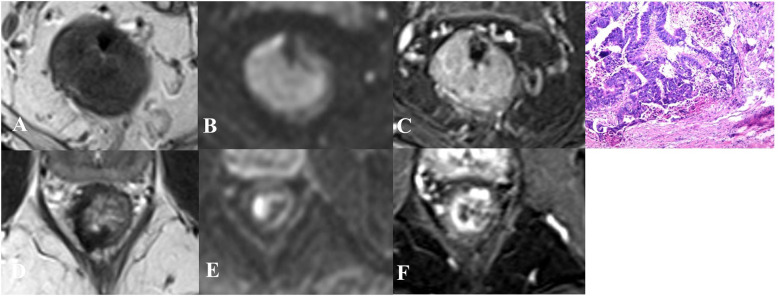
Imaging and pathological findings in a 71-year-old male with low rectal adenocarcinoma. (A–C) Baseline axial T2-weighted imaging, diffusion-weighted imaging (DWI), and contrast-enhanced MRI show a tumor occupying &gt;3/4 of the bowel circumference, characterized by intermediate T2 signal, restricted diffusion, and contrast enhancement. (D–F) After neoadjuvant chemoradiotherapy, the lesion area exhibits thickened, hypointense changes on T2-weighted imaging, persistent restricted diffusion on DWI, and rigid, thickened enhancement on contrast- enhanced imaging. (G) Postoperative pathological examination following total mesorectal excision confirmed the presence of residual tumor tissue within the submucosa and muscularis propria.
